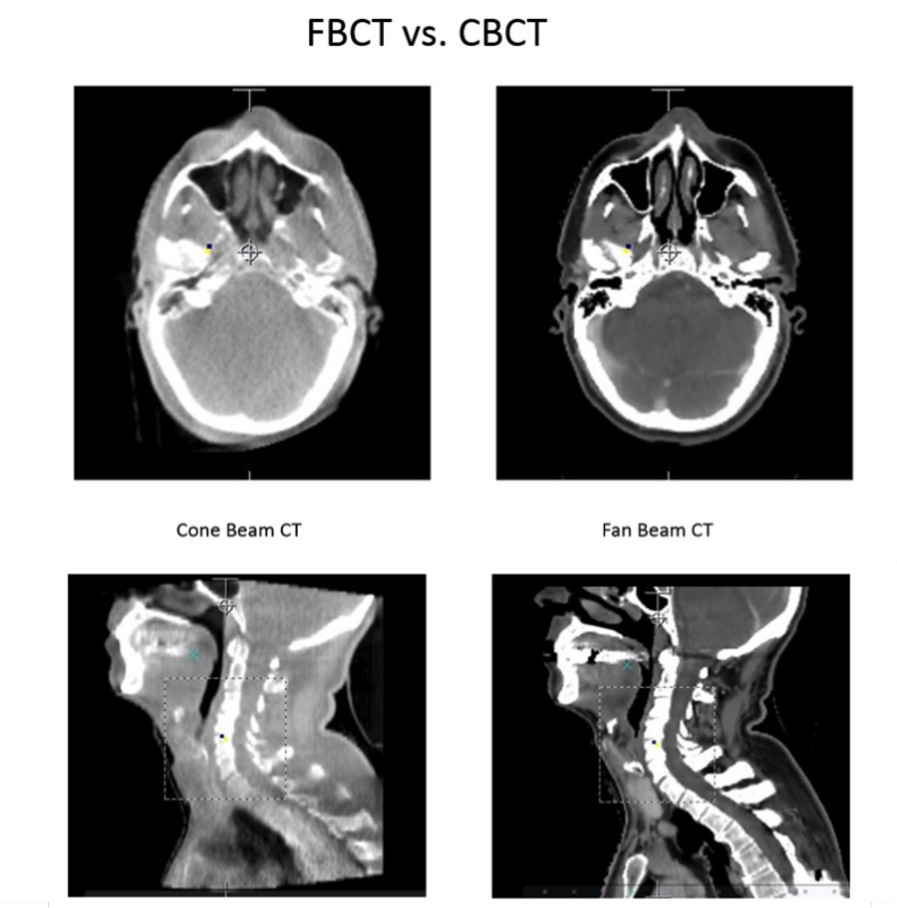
FBCT vs CBCT Cone beam CT (left) vs. fan beam CT (right) of head and neck IGRT in axial and sagittal orientation.
